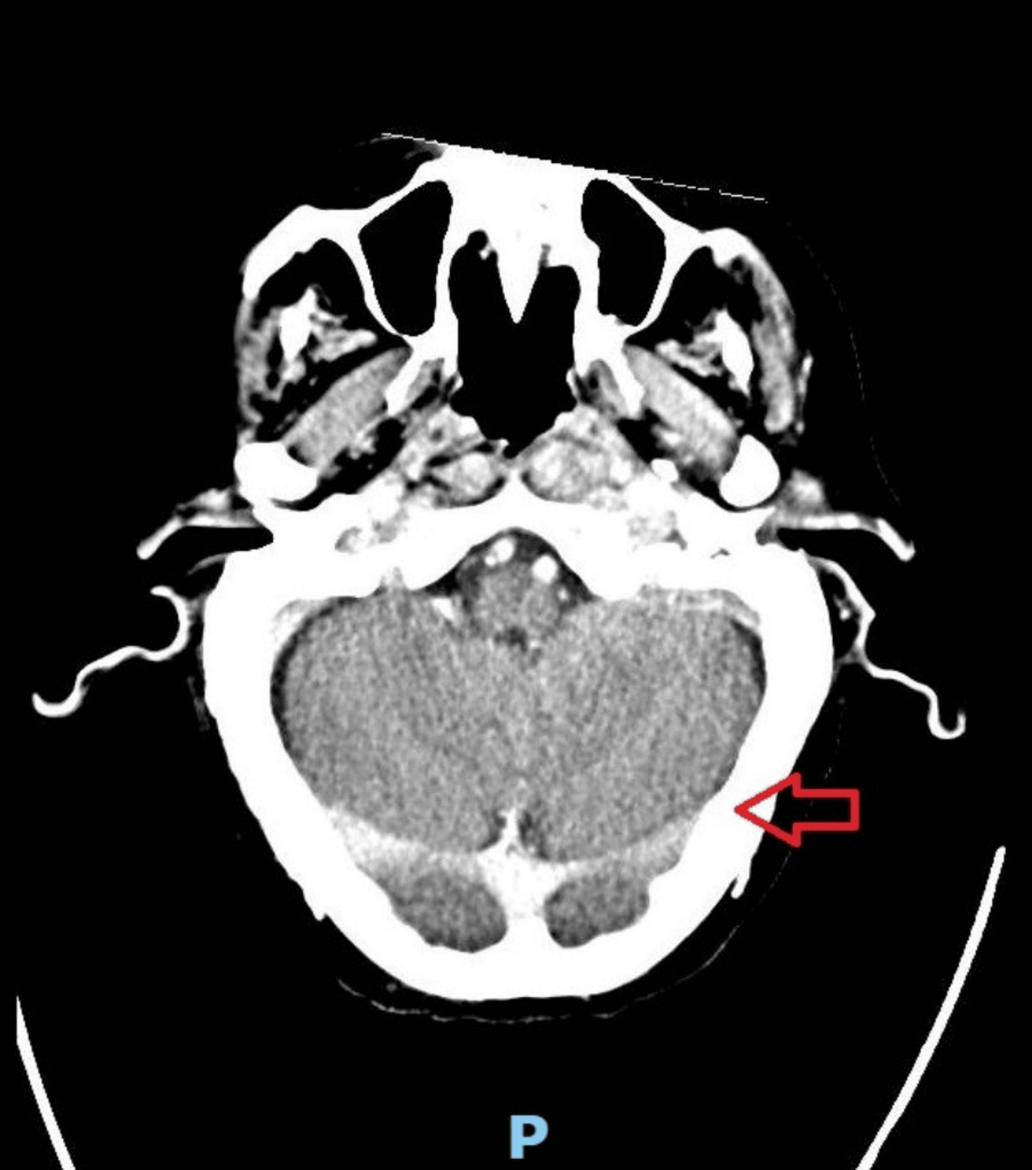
CT head; left sigmoid sinus thrombosis, secondary to the extension and progression of the disease
